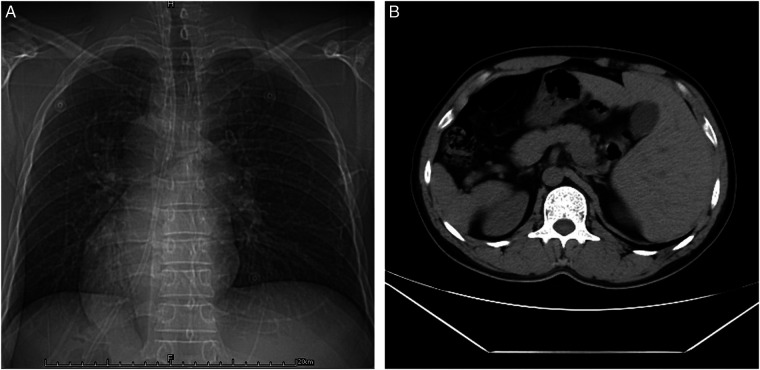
CT confirmed the patient with SIT. (A) Chest CT presented a right-sided view of the heart. (B) Abdominal CT suggested left–right viscera inversion.
